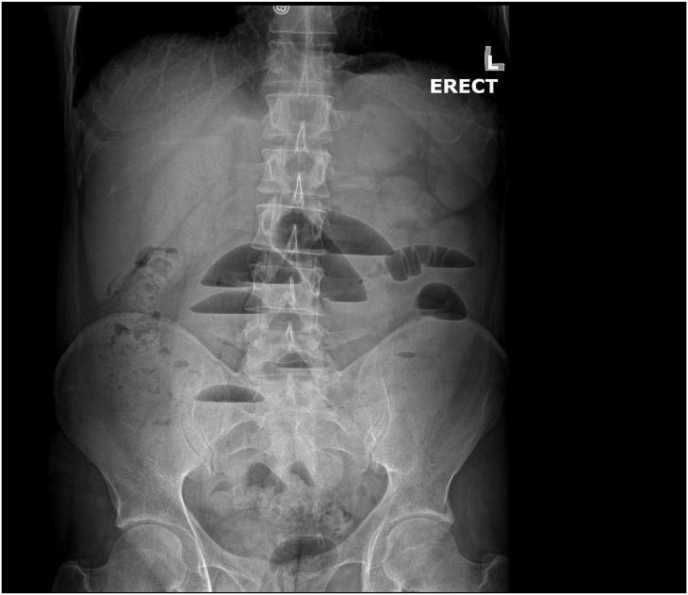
An abdominal x-ray divulging multiple air-fluid levels within the colon, thus alluding to an obstructive etiology.
